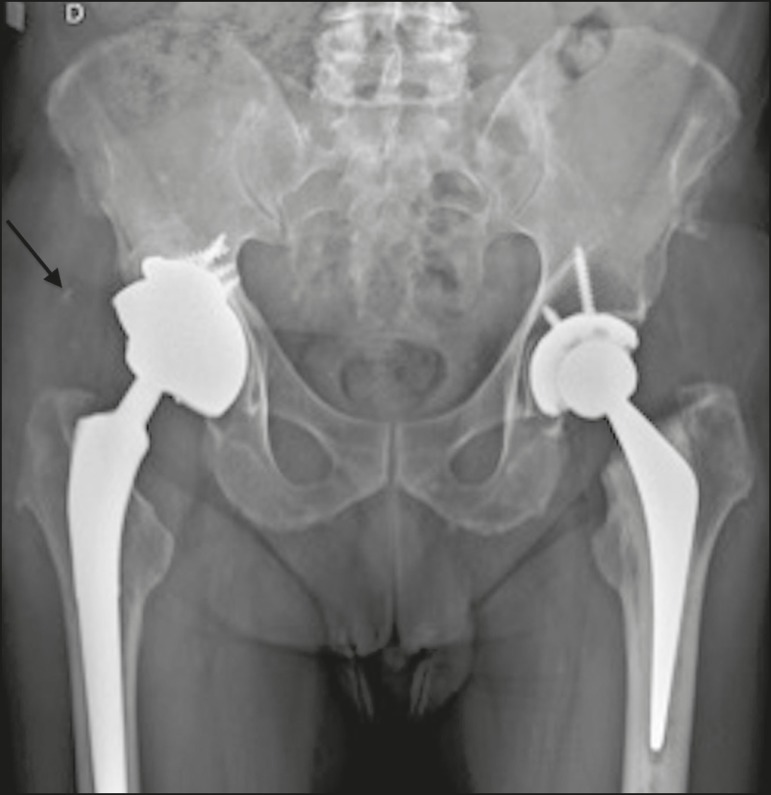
Anteroposterior X-ray of the right hip with grade I heterotopic ossification in the projection of lateral soft tissues on the right (arrow). Total hip arthroplasty in which the acetabular component of the prosthesis was affixed with screws, with no signs of loosening.
